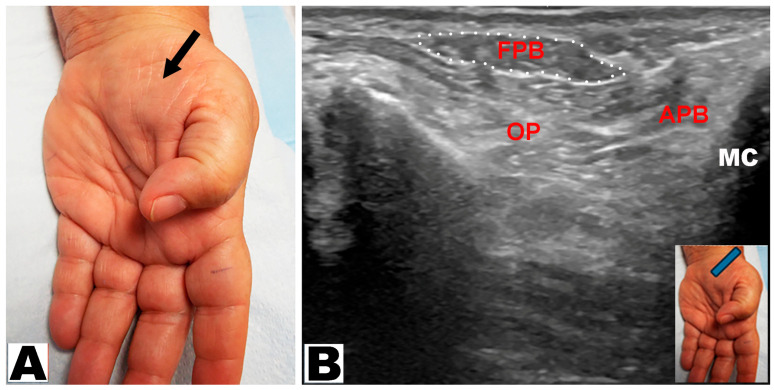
(A) Partial thenar atrophy: the flexor pollicis brevis muscle is visible (arrow) with atrophy of the abductor pollicis brevis muscle. (B) Ultrasound depicts partial thenar atrophy. The intact flexor pollicis brevis (FPB) muscle is encircled. APB: abductor pollicis brevis muscle. OP: opponens pollicis muscle. MC: metacarpal.
